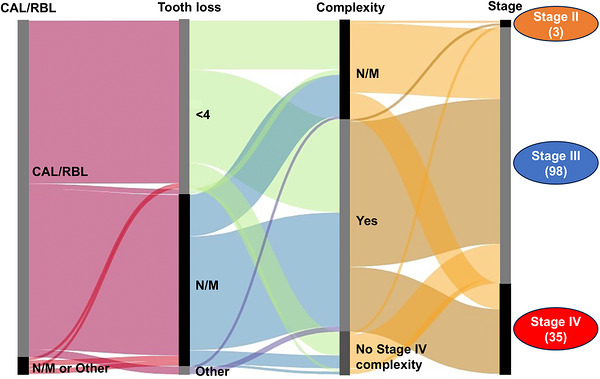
Alluvial plot for periodontitis stage (Case 2, Question 3, *n* = 136). CAL, clinical attachment loss; RBL, radiographic bone loss; N/M, not mentioned; Other included 1) CAL < 4 mm, multiple sites, or middle third, and 2) RBL coronal 1/3 or apical 1/3.
